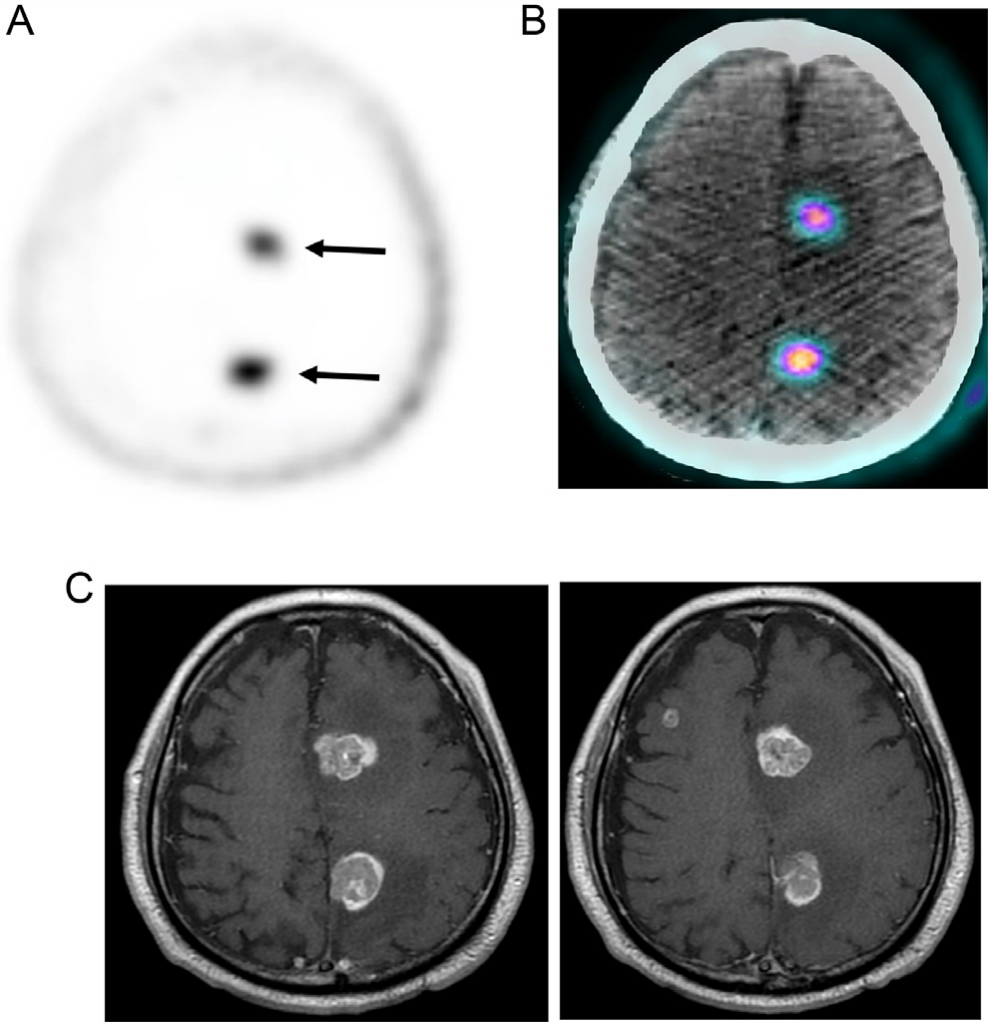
(A) Baseline axial PSMA PET brain lesions with SUV 5.0 and 7.8 and (B) PET/CT fusion image. (C) Brain MRI T1 with contrast shows enhancing lesions.
